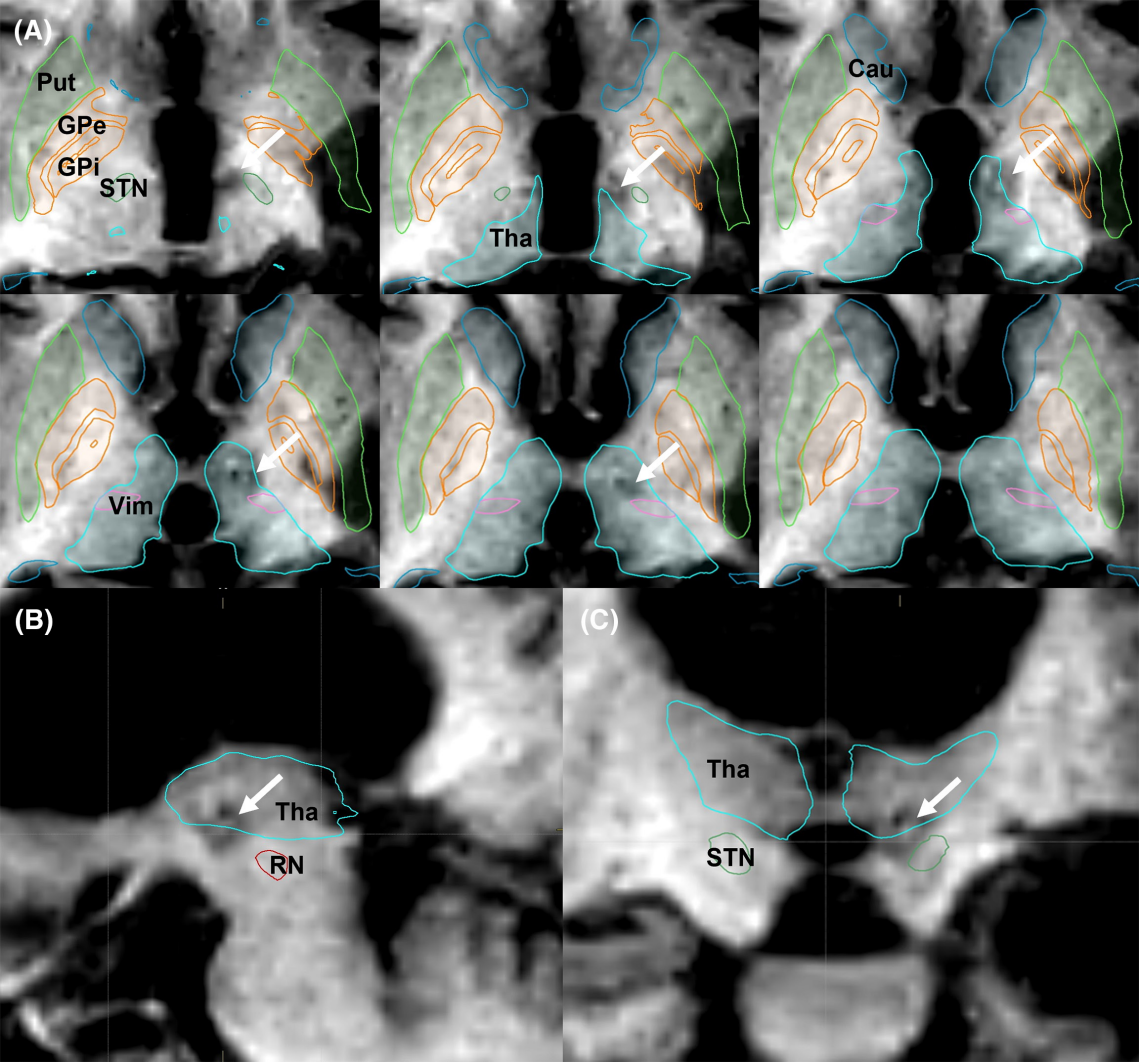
Postoperative magnetic resonance imaging (MRI) with anatomical mapping Brainlab elements. Postoperative T1‐weighted axial (A), sagittal (B), and coronal (C) MRI showing lesion (white arrow) and surrounding structures. Cau, caudate nucleus; GPi, globus pallidus internus; GPe, globus pallidus externus; Put, putamen; RN, red nucleus; STN, subthalamic nucleus; Tha, thalamus; Vim, ventral intermediate nucleus.
